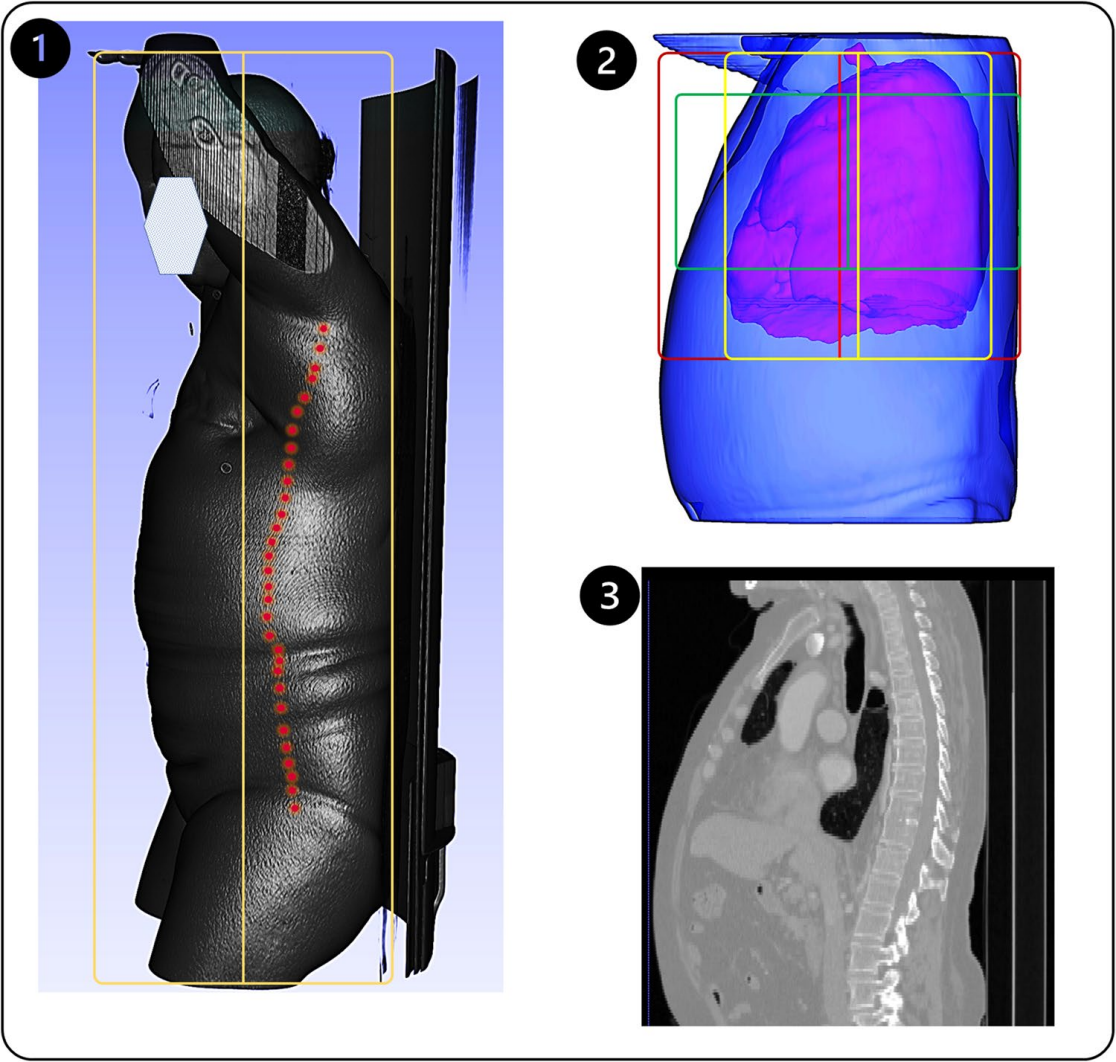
Effect of scan range on body centerline. (1) Lateral view of body contour (skin contour) from head to mid-thigh. The yellow box and line show the bounding box and the centerline can be detected by a visual camera with a zero-error accuracy. The red dots present the body centerline at each axial position. (2) A male patient chest 3D rendered image body contour (cyan) and lung segmentation (red). Three bounding boxes and centerlines are visualized. The red one shows body B.Box and centerline for chest CT typical scan range. The yellow one shows the lung B.Box and centerline for chest scan range. The green one shows the B.Box and centerline for typical cardiac scan range. (3) A coronal slice of the same patient shown in (2)
